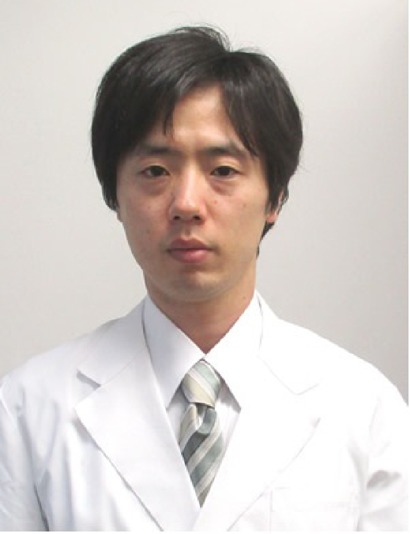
After graduating from university in 2008, Junichi Ooka went into the path of cardiology after 2 years of residency. After studying general cardiology for 3 years, he have conducted research activities focusing on echocardiography for 4 years at Kobe University.
